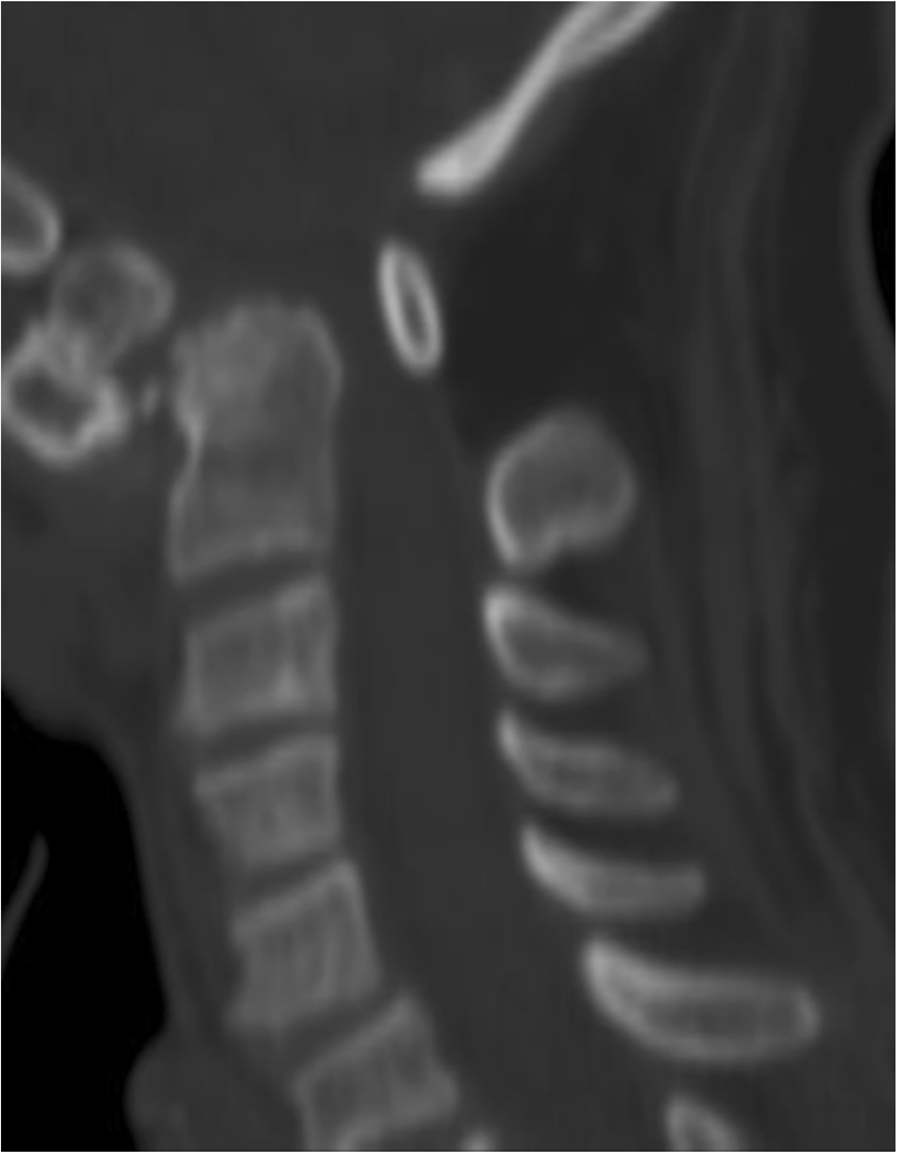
Sagittal cervical CT of a 68-year-old Down syndrome patient, showing the presence of an *os odontoideum* with atlanto-axial and atlanto-odontoid luxation; there is severe stenosis of the craniocervical junction, with space conflict in the bulbo-medullary junction
